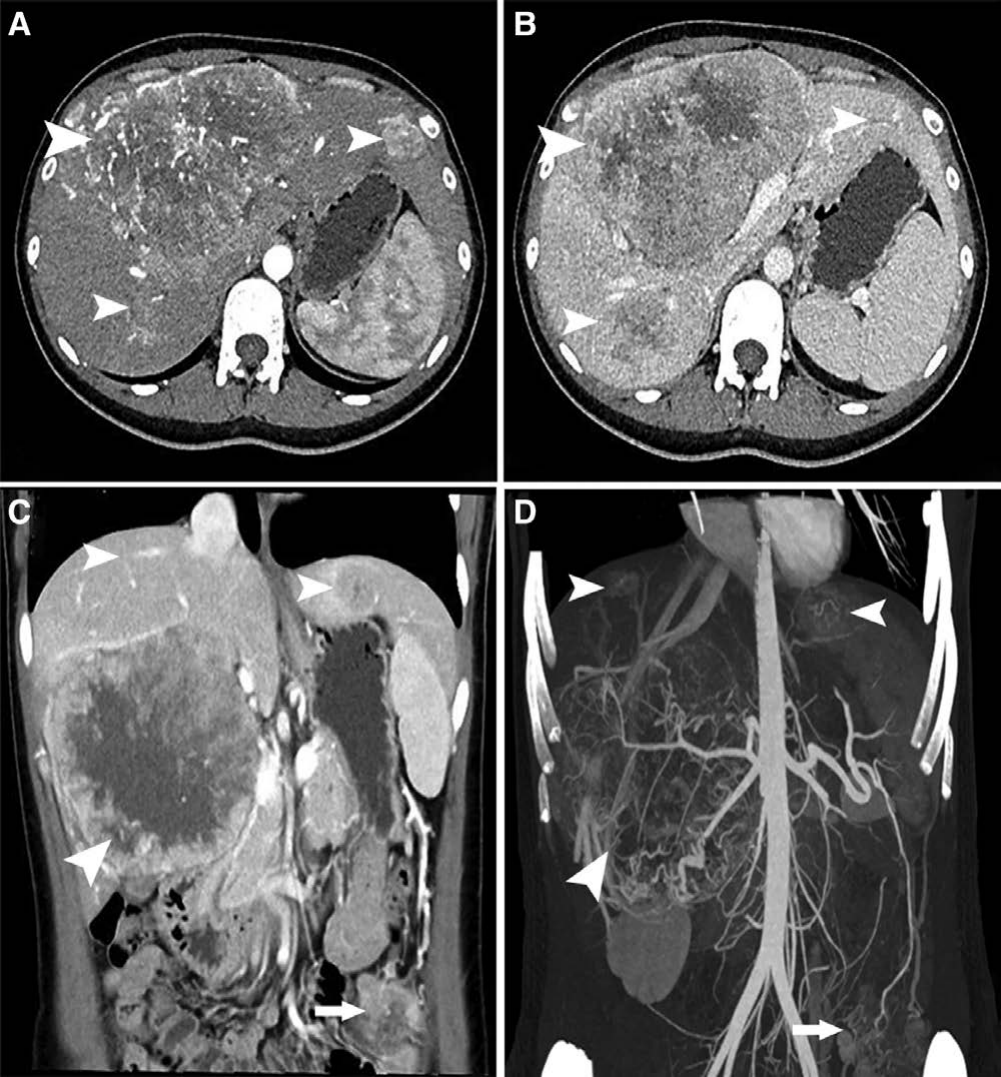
Multiphase contrast-enhanced CT of the liver tumor (arrowheads) and intra-abdominal metastases (arrows). (A) contrast-enhanced CT image in the arterial phase; (B) contrast-enhanced CT image in the venous phase; (C) coronal reformatted CT image; (D) CT vascular reconstruction image. CT = computed tomography.
